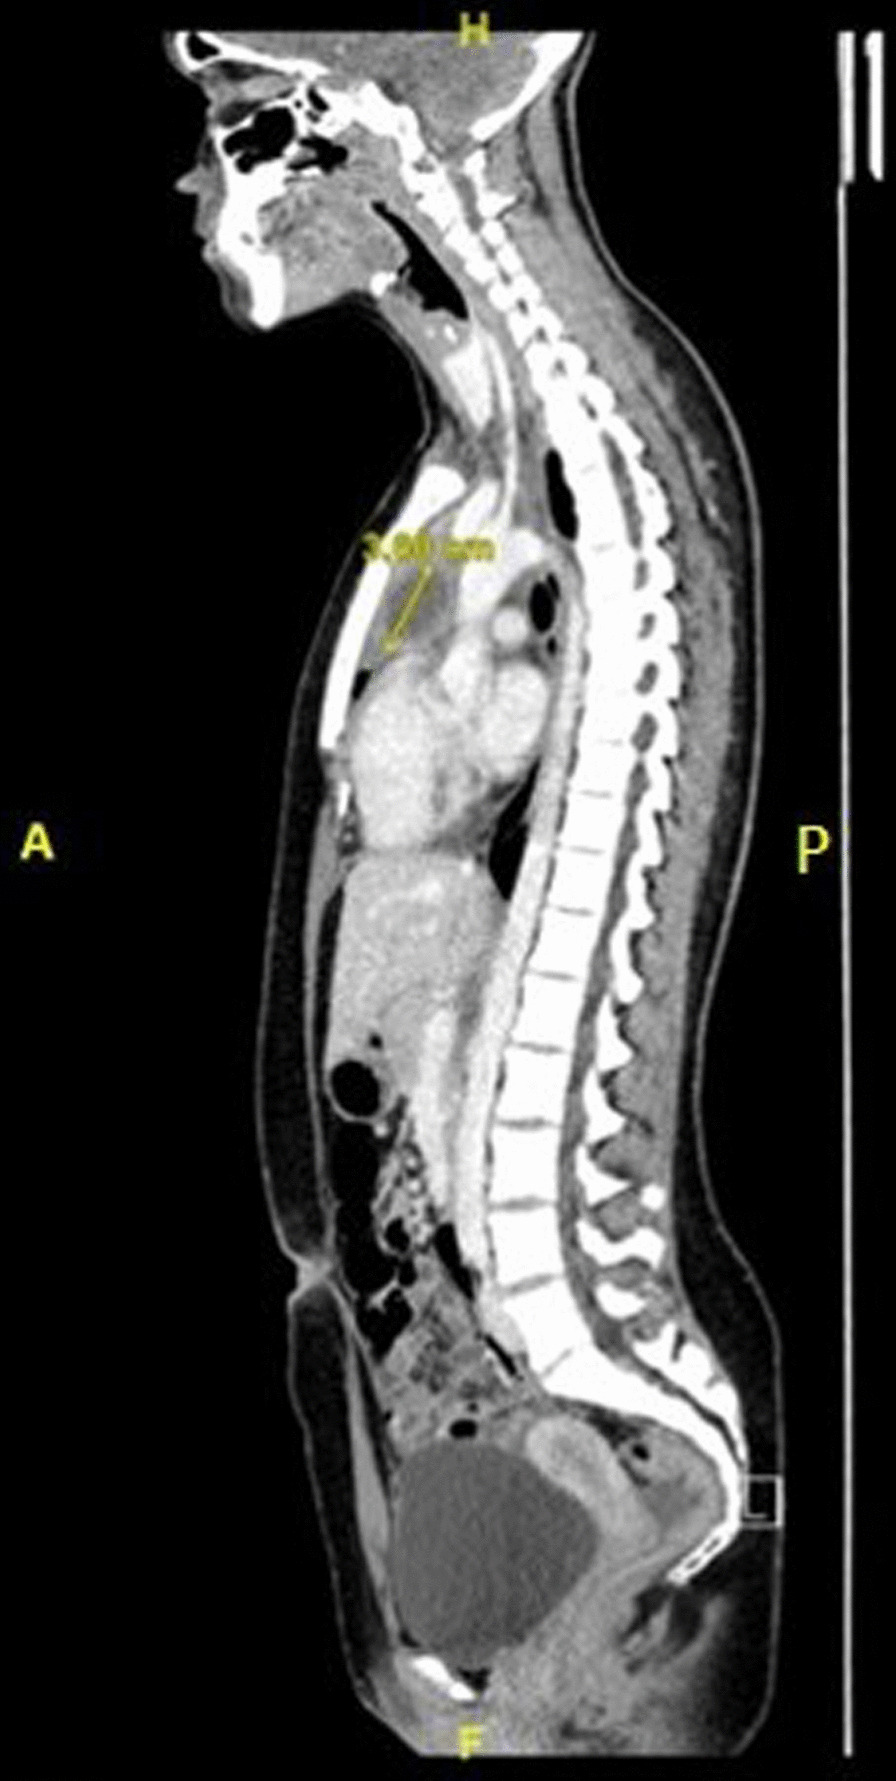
Sagittal plane of CT. This image highlights the presence of a 3.3cm × 3.8 cm cystic mass in the anterior mediastinum
